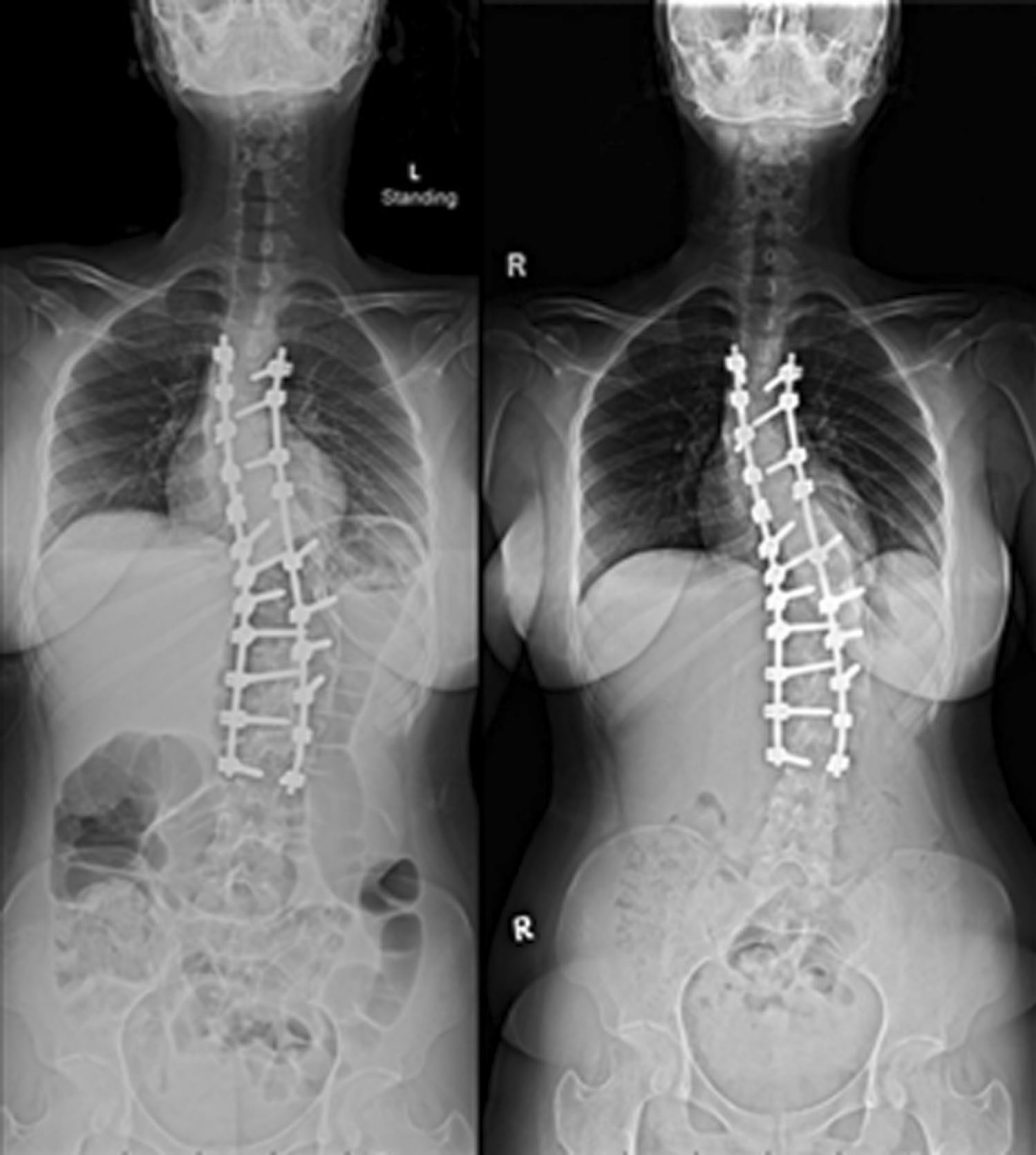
An example of spontaneous correction of PSI. (Left) Immediate postoperative standing whole-spine frontal radiograph of an AIS patient revealed PSI. (Right) Whole-spine frontal radiograph at 1 year follow-up in the same patient revealed spontaneous correction of PSI
